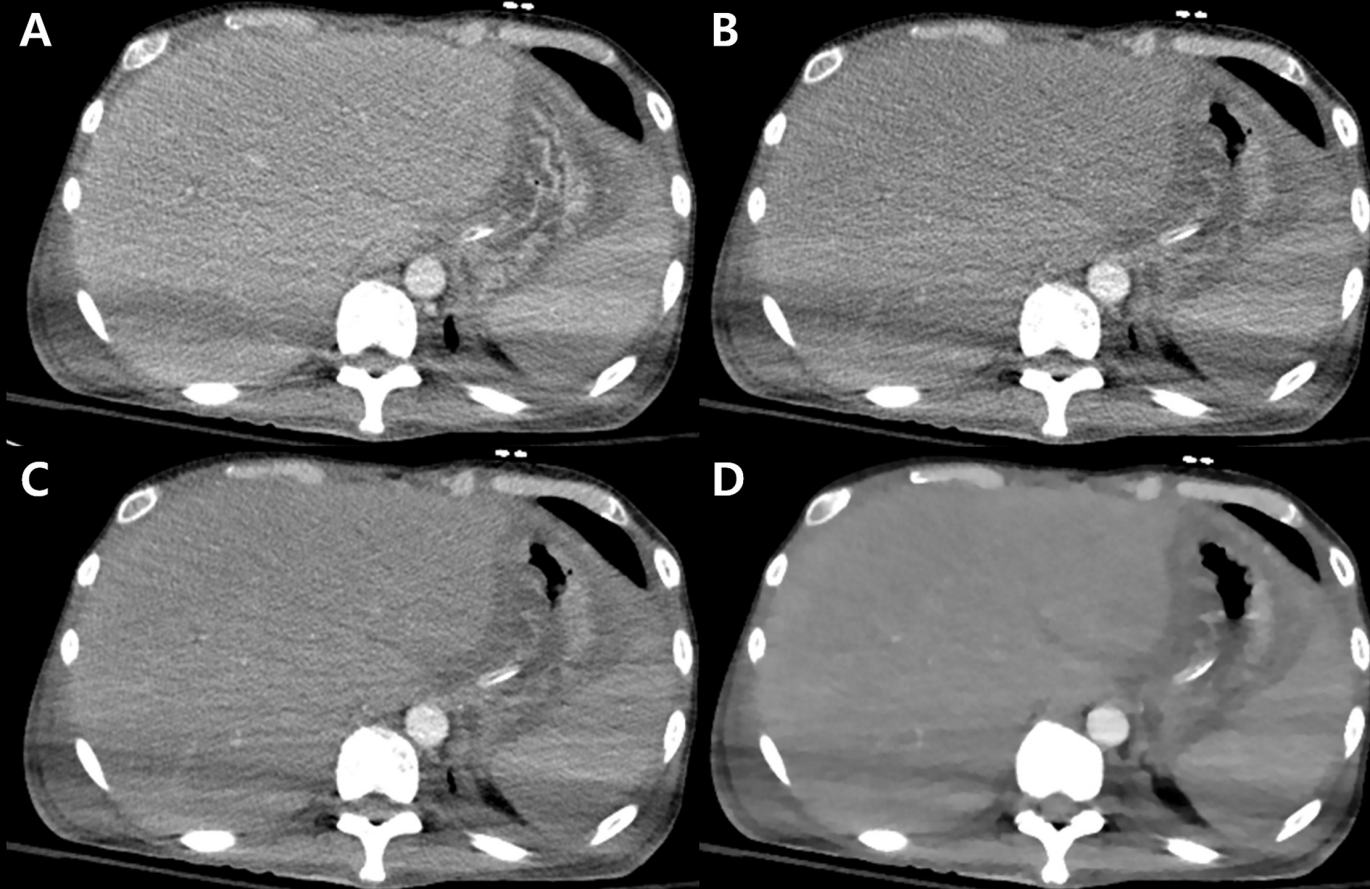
Multidetector CT images (A: standard-dose CT image; B-D: reduced-dose CT images) of a 45-year-old male patient with his two arms positioned on his sides. The section thickness was 3 mm. The window width and level was fixed at 350 and 50, respectively throughout the reconstructed image set. (A) The standard-dose CT image with filtered back projection (FBP) reconstruction shows the presence of beam hardening artifacts associated with the arms-down position. (B) The reduced-dose CT image with FBP reconstruction shows more prominent hypodense and hyperdense artifacts in the liver and spleen compared with (A). (C) A reduced-dose CT image with hybrid iterative reconstruction (iDose level 4) had similar or slightly less beam hardening artifacts than (A) while image quality was preserved. (D) A reduced-dose CT image with iterative model reconstruction (IMR level 3) shows less beam hardening artifacts than (A-C). However, IMR negatively affected the overall image quality due to prominent artificial texture and poor margin sharpness.
